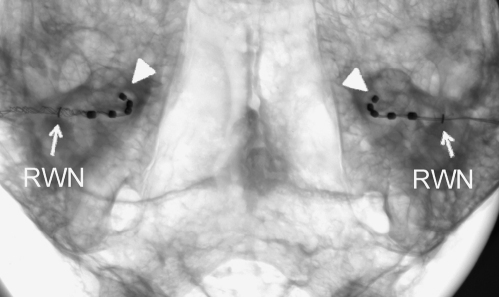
High resolution micro-focus radiograph in anterior–posterior view of a ferret skull showing bilateral intracochlear arrays inserted to an even depth into the basal turn of both cochleas relative to the round window niche (RWN) marked with a fine wire. The apical electrode in the electrode array is marked with an arrowhead.
